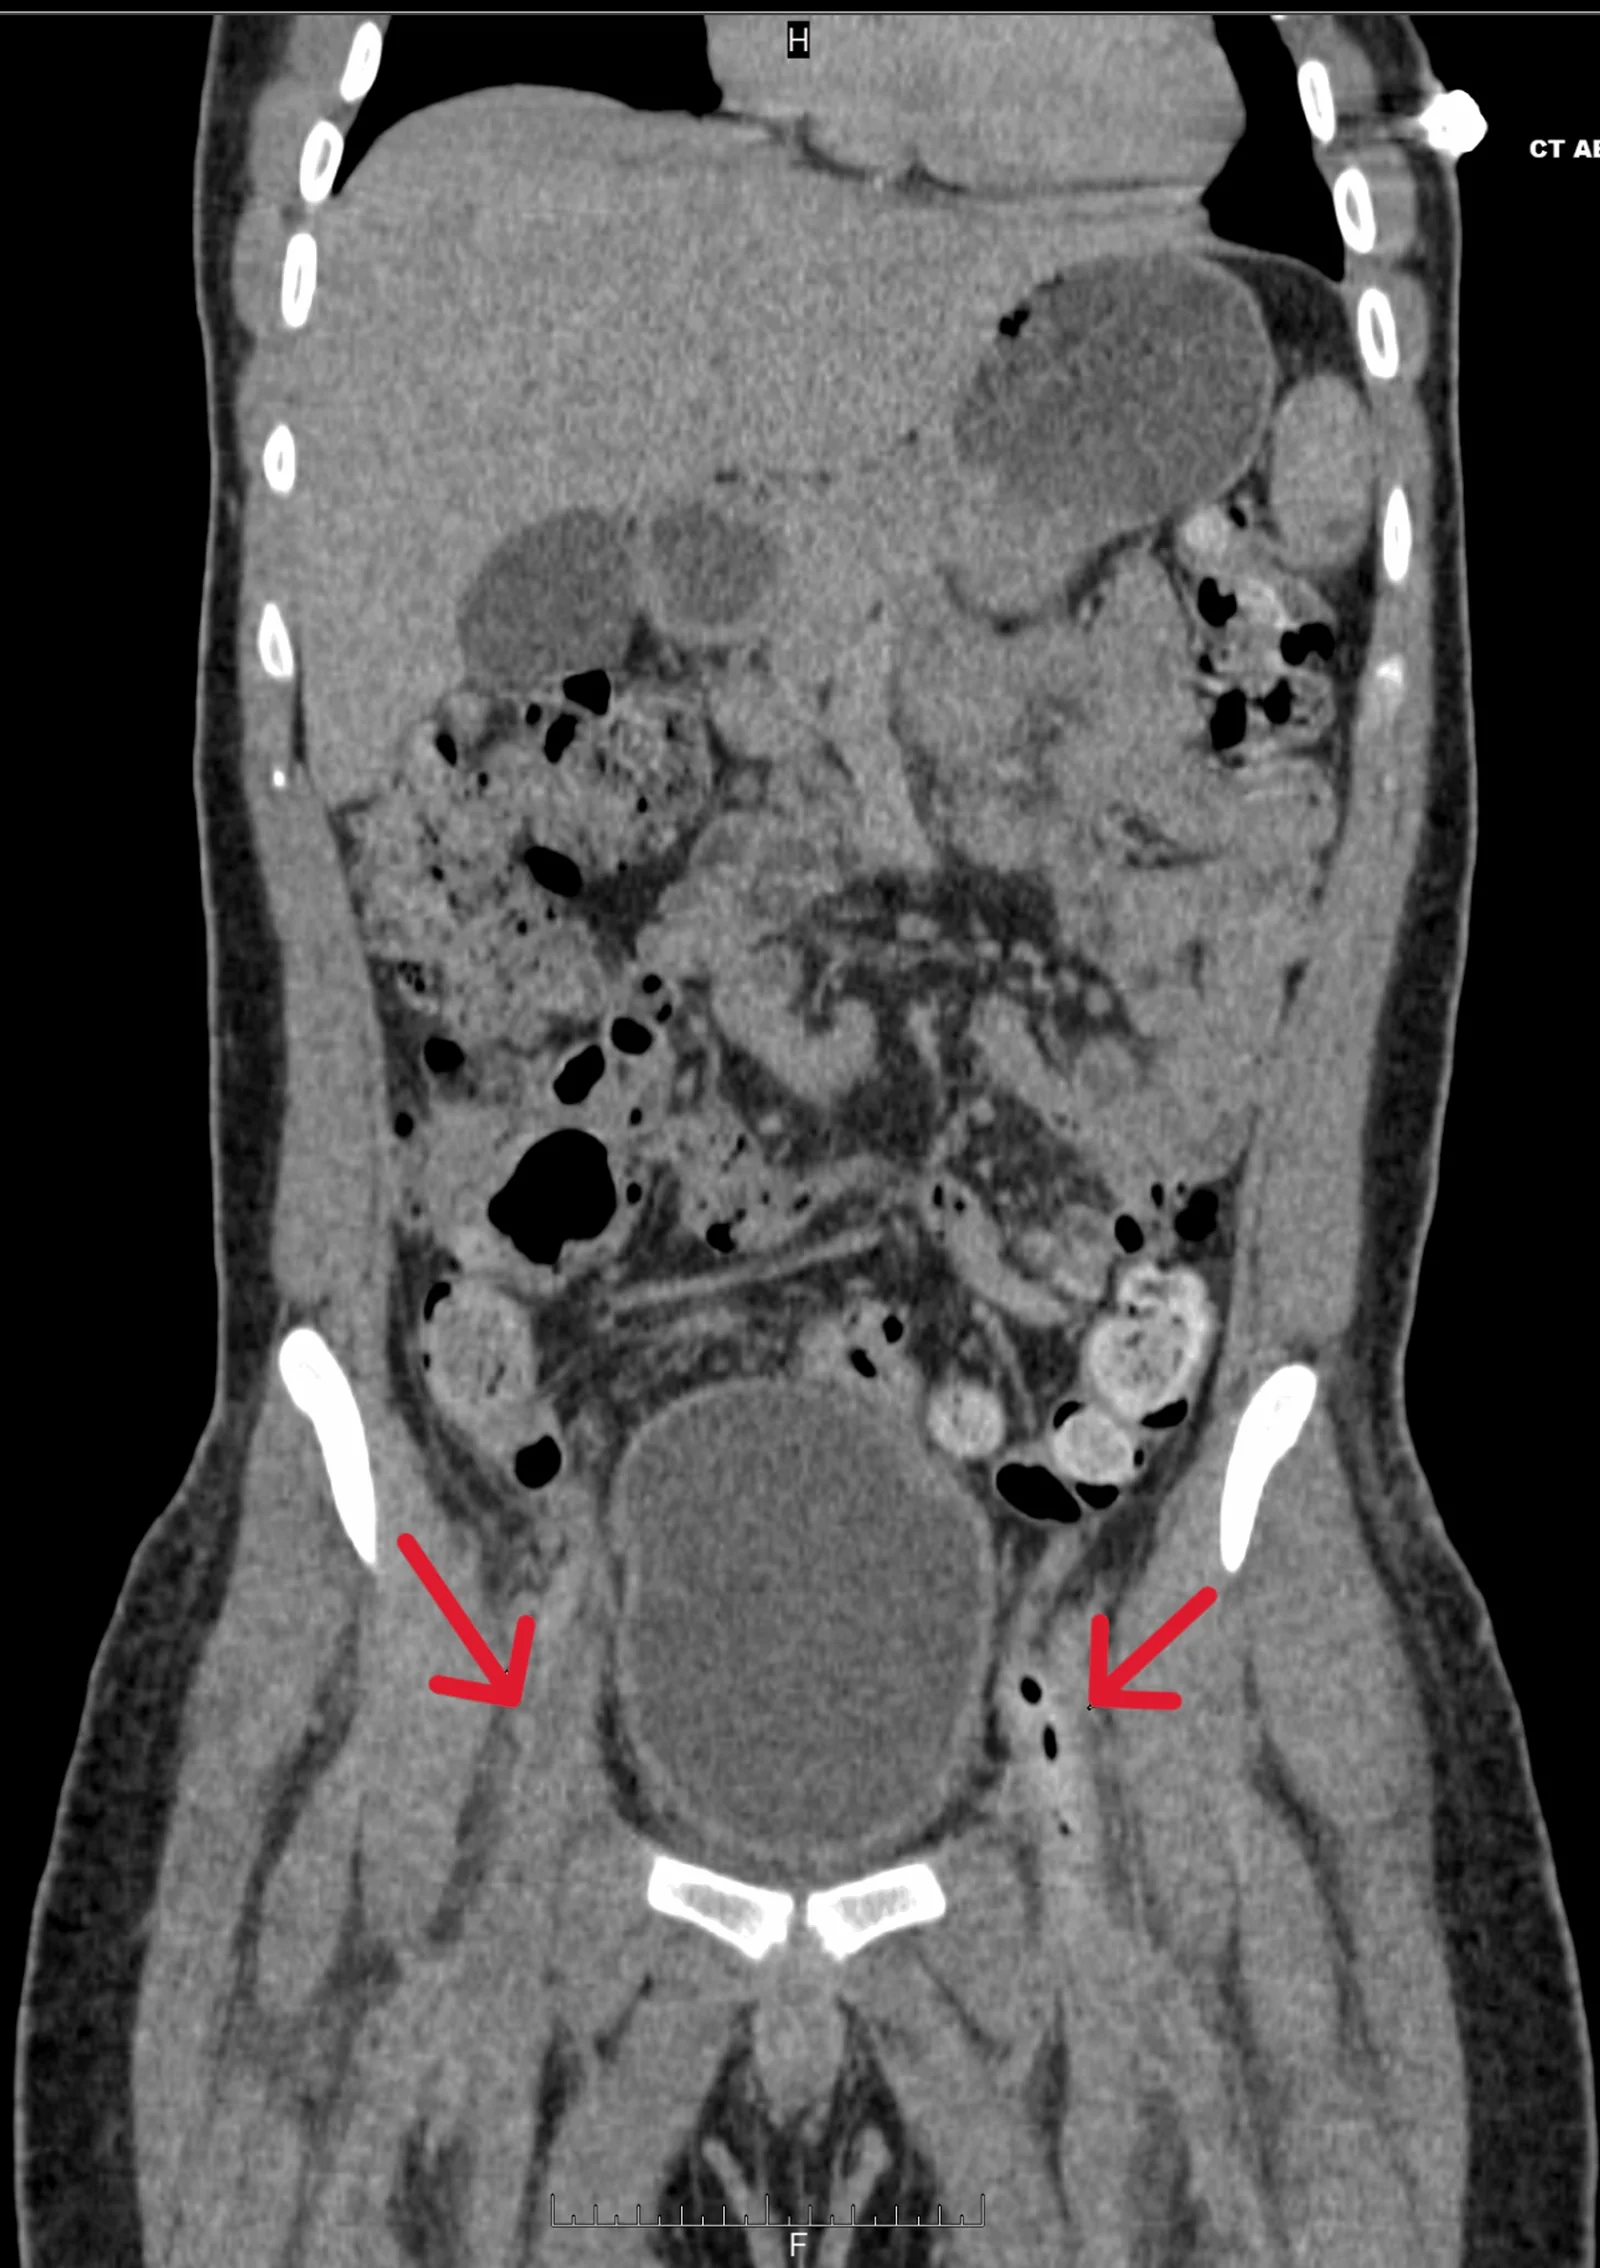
Coronal computed tomography angiography showing bilateral iliac/common femoral venous involvement (red arrows)
